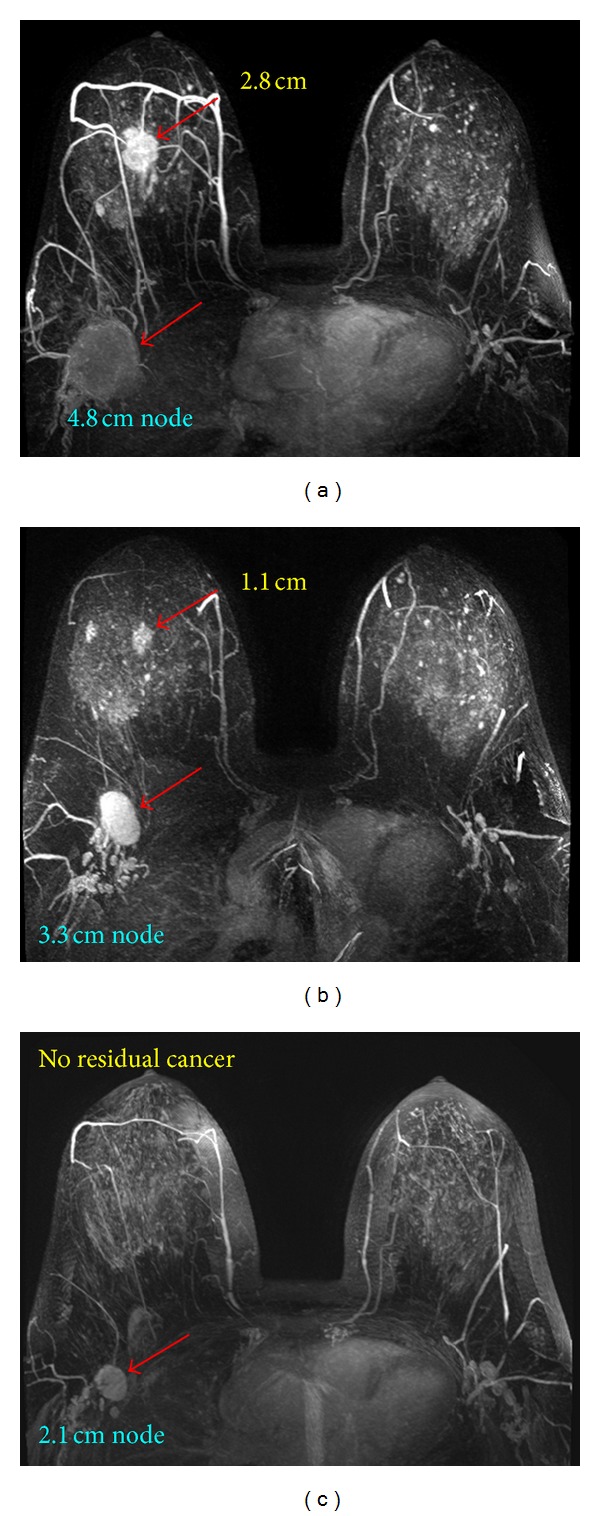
A 48-year-old patient with a mass lesion (invasive ductal cancer) in the right breast and an enlarged lymph node in the axilla. From (a) to (c), the maximum intensity projection (MIP) images of pretreatment, F/U-1, and F/U-2 MRI are shown. The size of the primary tumor in the breast is 2.8 cm before treatment, which shrinks down to 1.1 cm in F/U-1 and reaches a complete response in F/U-2 after completing NAC. The node is also responding well and shows size shrinkage from 4.8 cm before treatment to 3.3 cm in F/U-1 and to 2.1 cm in F/U-2. In addition to evaluating the response of primary tumor, MRI can also be used to evaluate the response in the nodes. This patient is confirmed to reach pCR in the post-NAC pathological examination, with one positive node.
